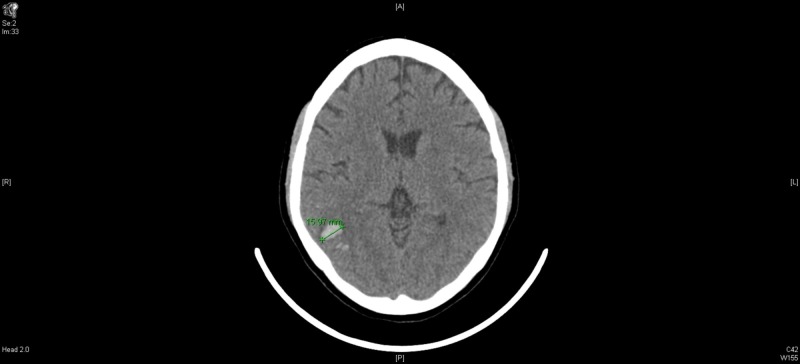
Computed tomography (CT) head without contrast, day 1.
